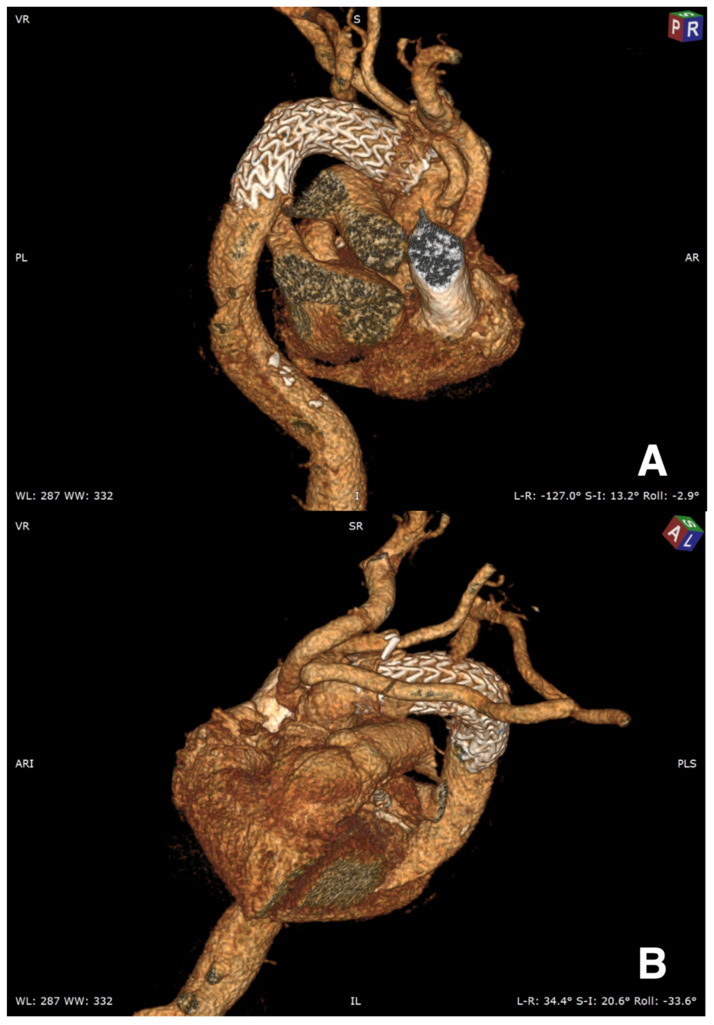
Postoperative 3D CT scan reconstruction. (A) Zone 1 Teflon-reinforced distal anastomosis. (B) Supra-aortic vessels reconstruction with left subclavian artery extra-anatomic implant.
